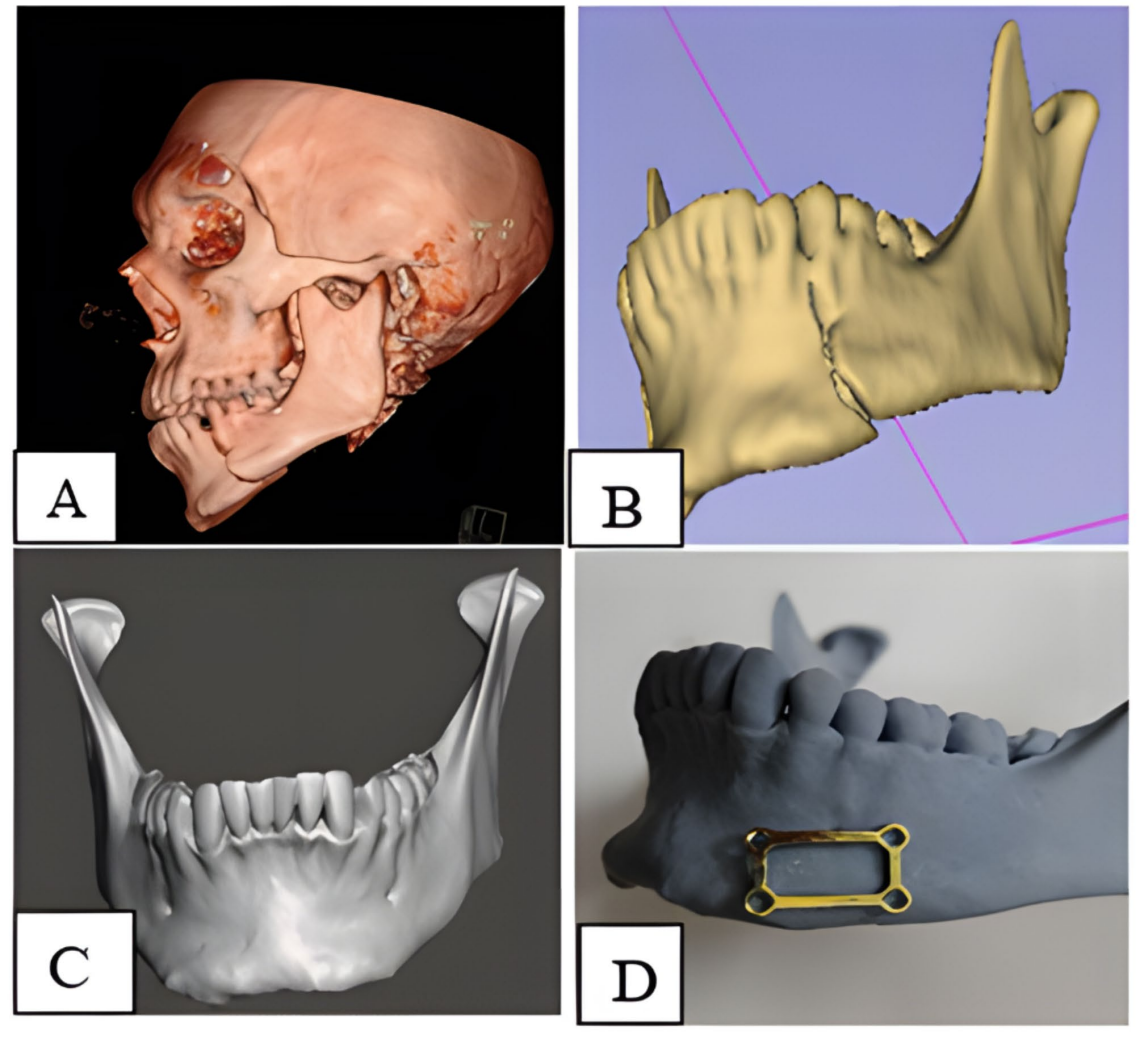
Preoperative surgical planning. (A) 3D reconstructed image from CT. (B) segmented mandible from CT. (C) virtually reduced mandibular fracture. (D) printed 3D model with prebent miniplate
